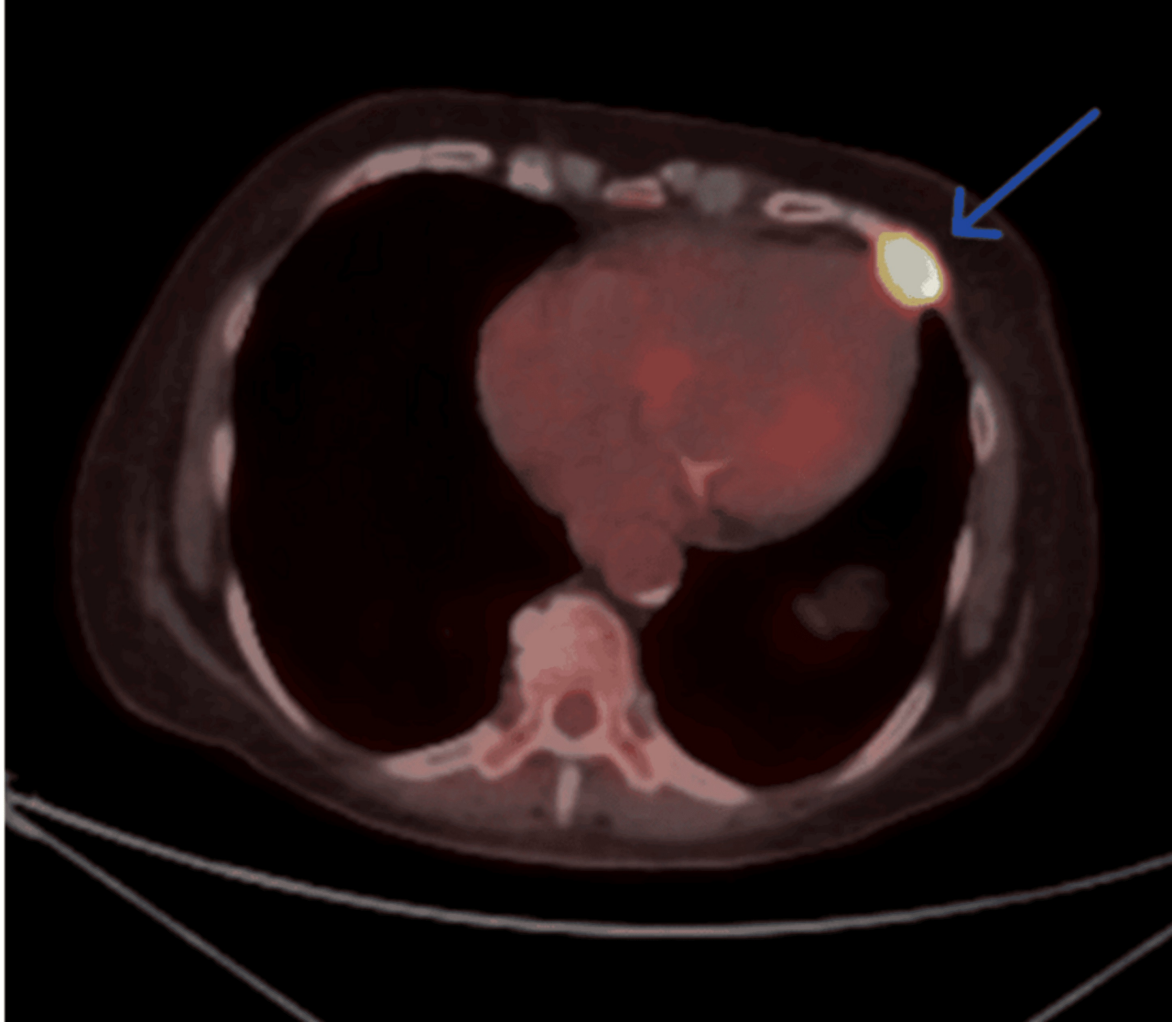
Axial PET-CT image showing FDG-avid lytic lesion in the left anterior fifth rib. Axial view of PET-CT revealed a fluorodeoxyglucose (FDG)-avid lytic lesion in the left anterior fifth rib with increased radiotracer uptake, consistent with osseous metastasis from the primary basal cell carcinoma.
